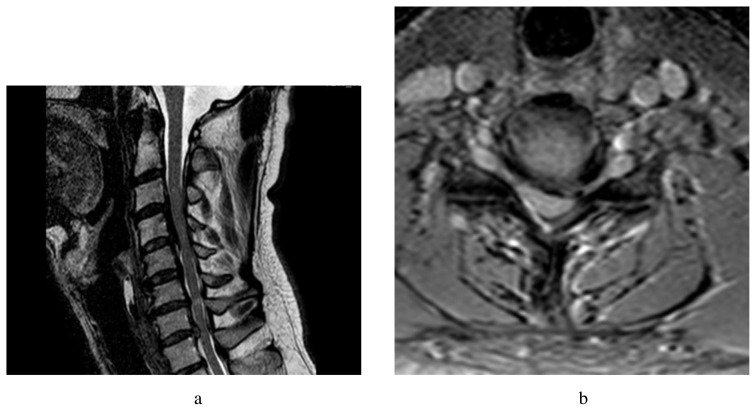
Postoperative sagittal T2 weighted MRI is demonstrating enlargement of the cervical spinal canal and relief of CSF passaged raw attention. B. Postoperative axial T2 weighted MRI is showing enlargement of the cervical spinal canal.
